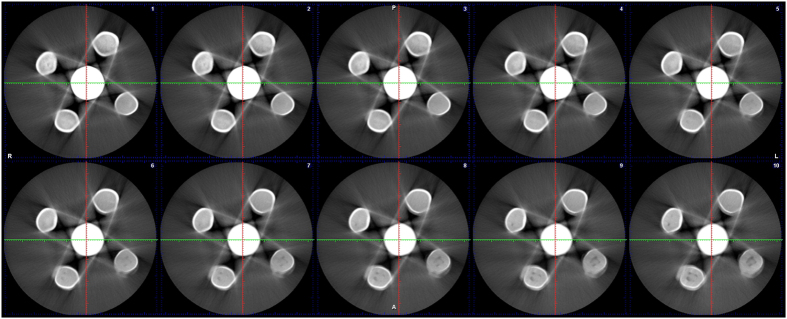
Ten two-dimensional CBCT images of one dental material with clear artefacts.
